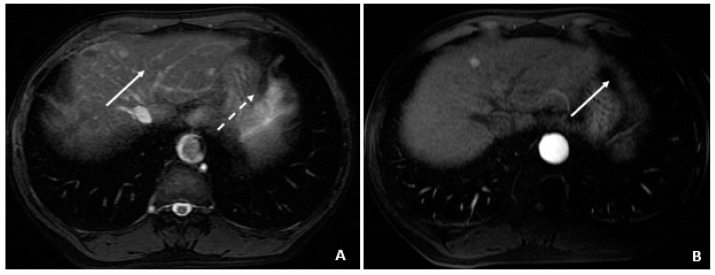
Initial MRI with normal AFP and elevated %L3 (July 2008). (A) The axial T2-weighted gradient echo image shows a small hyperintense lesion (arrow) in segment 4A. A second hyperintensity (dashed arrow) corresponds to a hemangioma. (B) The corresponding axial T1-weighted fat-suppressed arterial-phase postcontrast image shows hyperenhancement (arrow) in the lesion subsequently found to be a small HCC, although currently without associated features to clinch the diagnosis. Without washout, capsule appearance, or other typical HCC findings, the lesion is indeterminate.
